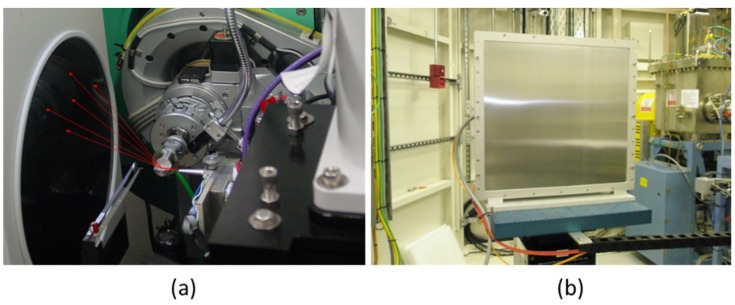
(a) MAR345 detector at SSRL beamline 11-3. X-ray trajectory is represented by red arrows; (b) Pixium RF4343 detector, covered with Al front plate, at Diamond Light Source beamline I12.
